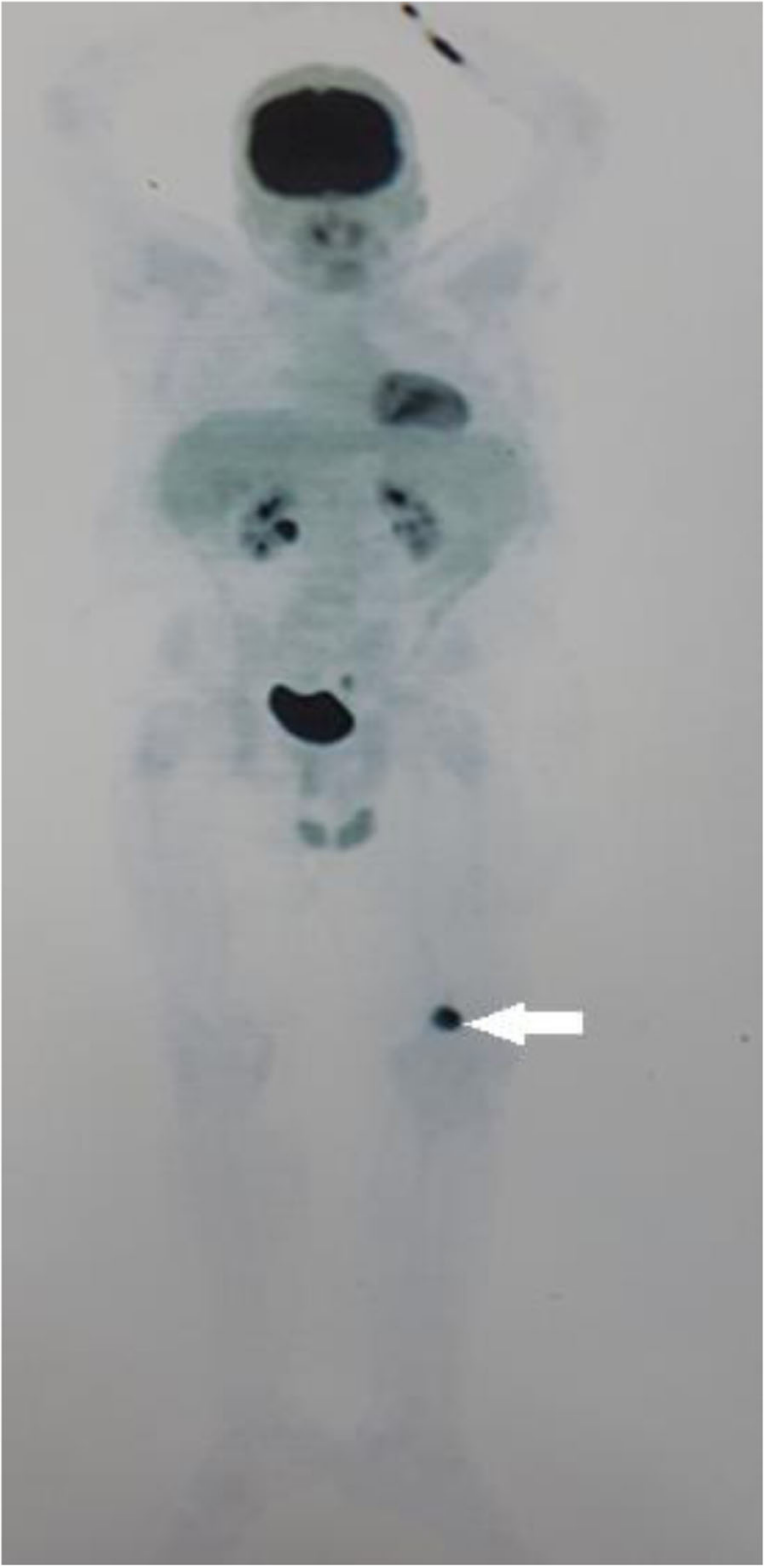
18F–FDG PET: a hypermetabolic lesion in medial aspect of the left distal femur (arrow)
